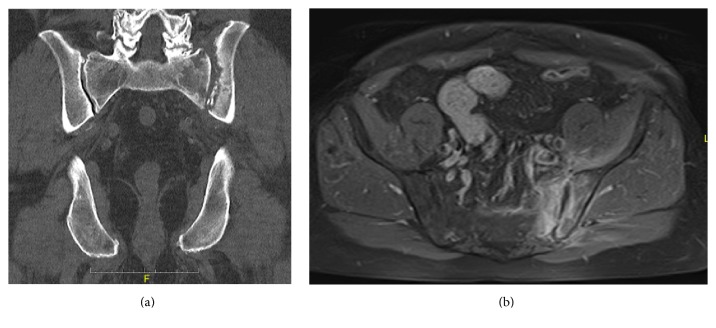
Control CT and MRI of the pelvis 2 months later: osteolytic destruction of the massa lateralis with fluid around the piriformis muscle and the ilium bone.
